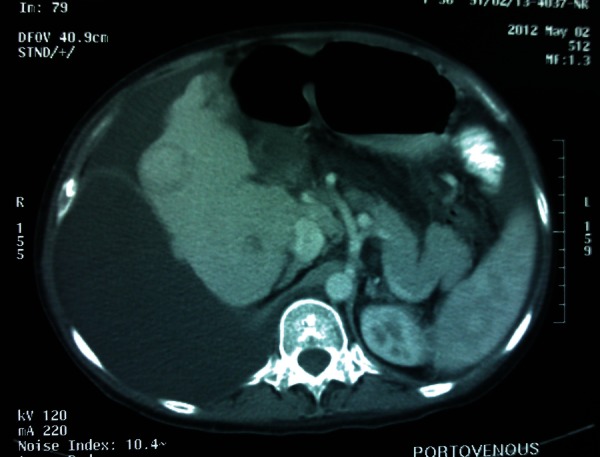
CT Scan of the Liver Shows a Well-Defined Hypoechoic Lesion in Right Lobe, 3X1.9X1 cm.
